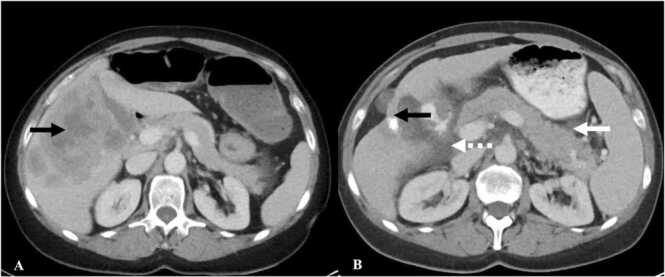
Immune-mediated pancreatitis in a 55-year-old man with melanoma on Pembrolizumab. A. Contrast-enhanced axial CT abdomen image shows numerous hepatic metastases (black arrow) with normal appearing pancreas. B. Contrast-enhanced axial CT abdomen image after the initiation of Pembrolizumab shows decrease in the hepatic metastases with liver capsule retraction (black arrow). Note that the pancreas is now bulky with peripancreatic stranding (white arrow) and fluid in the pancreaticoduodenal groove (dashed white arrow) suggesting pancreatitis.
